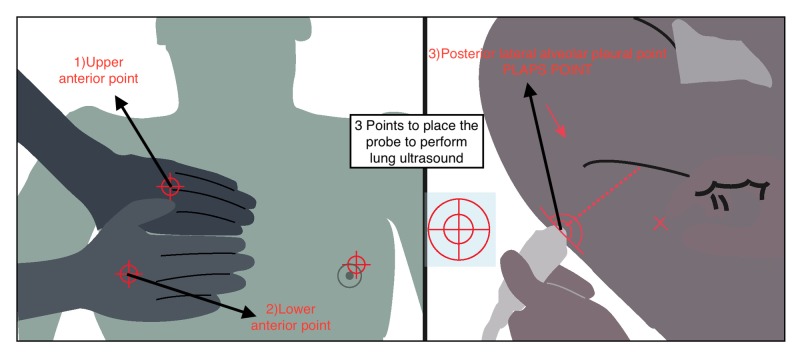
Three basic points where lung ultrasound should be performed
